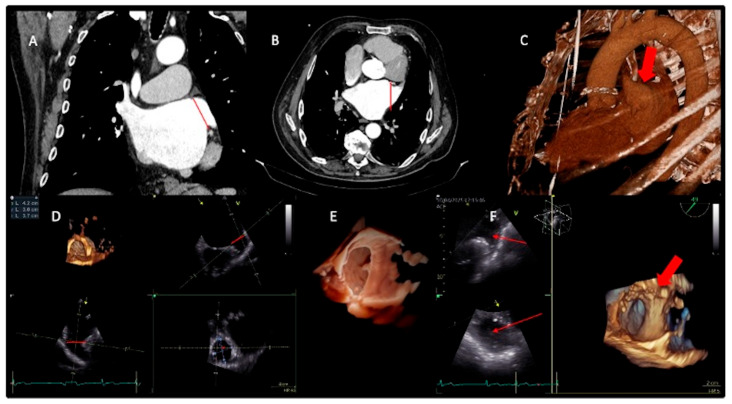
(A,B) CCT showing wide and shallow LAA (LAA orifice marked with a thin red line). (C) 3D CT reconstruction of LAA (red arrow pointing to the LAA). (D–F) 3D TEE reconstruction of large and shallow LAA (dimension of orifice 42 mm × 37 mm) (LAA orifice marked with a thin red line, red arrow marking the LAA).
